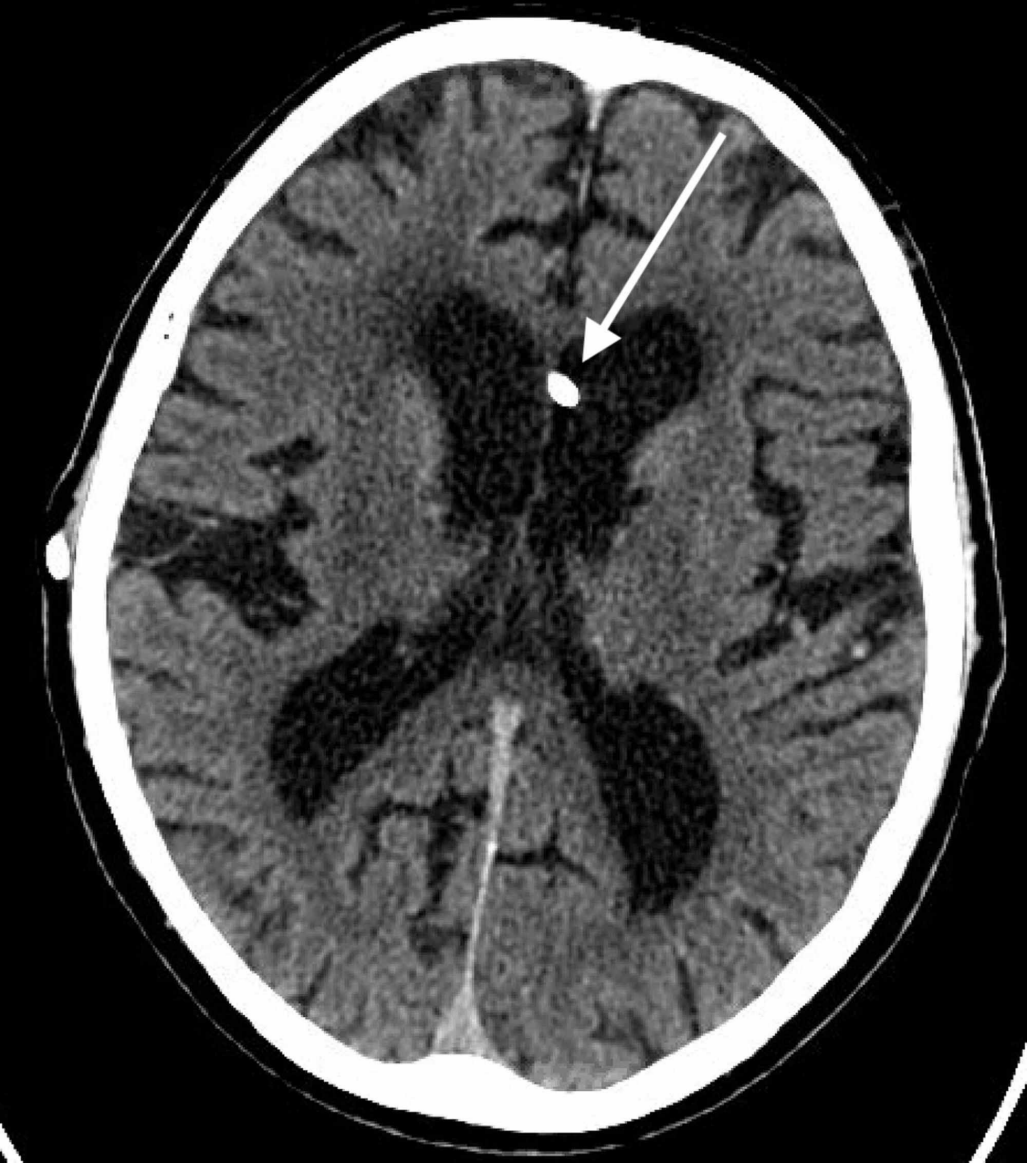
Computed tomography (CT) of the head without contrast performed during workup for the patient’s headaches shows tip of the proximal catheter within the left lateral ventricle.
